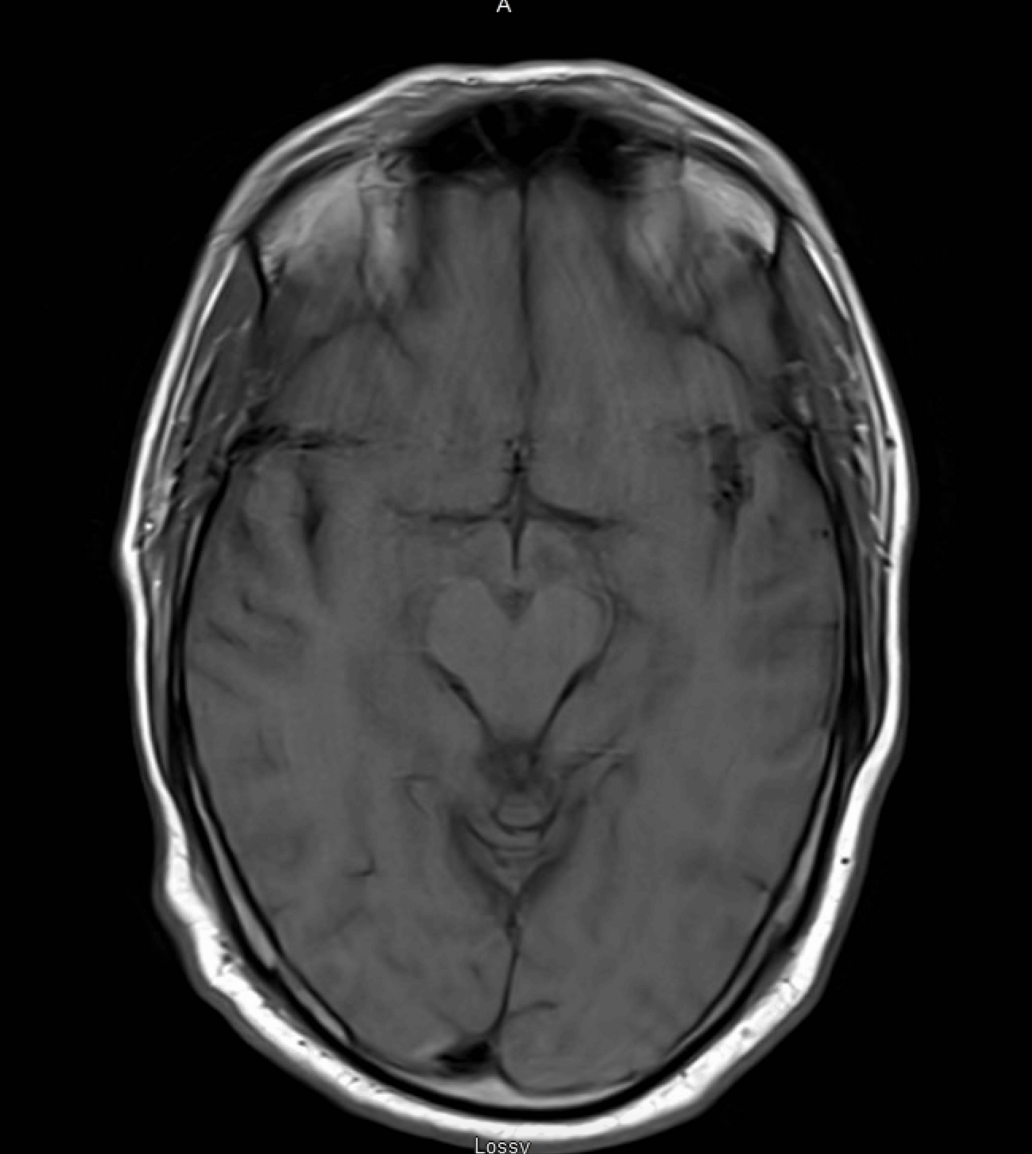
MRI showing no brain involvement
